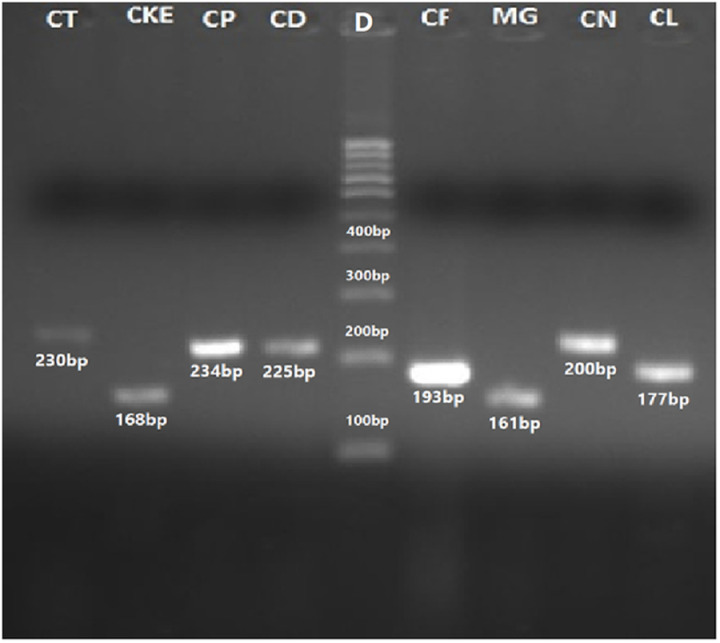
PCR amplified products of the PLB gene through specie-specific: CT = Candida tropicalis (230 bp), CKE = C. kefyr (168 bp), CP = C. parapsilosis (234 bp), CD = C. dubliniensis (225 bp), CF= C. famata (193 bp), MG= Meyerozyma guilliermondii (161 bp), CN= Cryptococcus neoformans (200 bp) CL= C. lusitaniae (177 bp) and D= 100 bp ladder (5).
